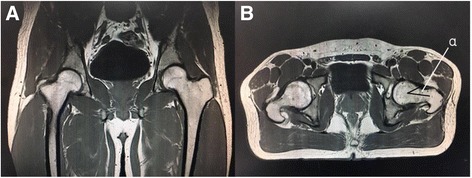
Differential diagnosis of intra-articular pathologies in MRI images. a Normal hip in coronal section. b Normal angle *α* showed in transverse section
